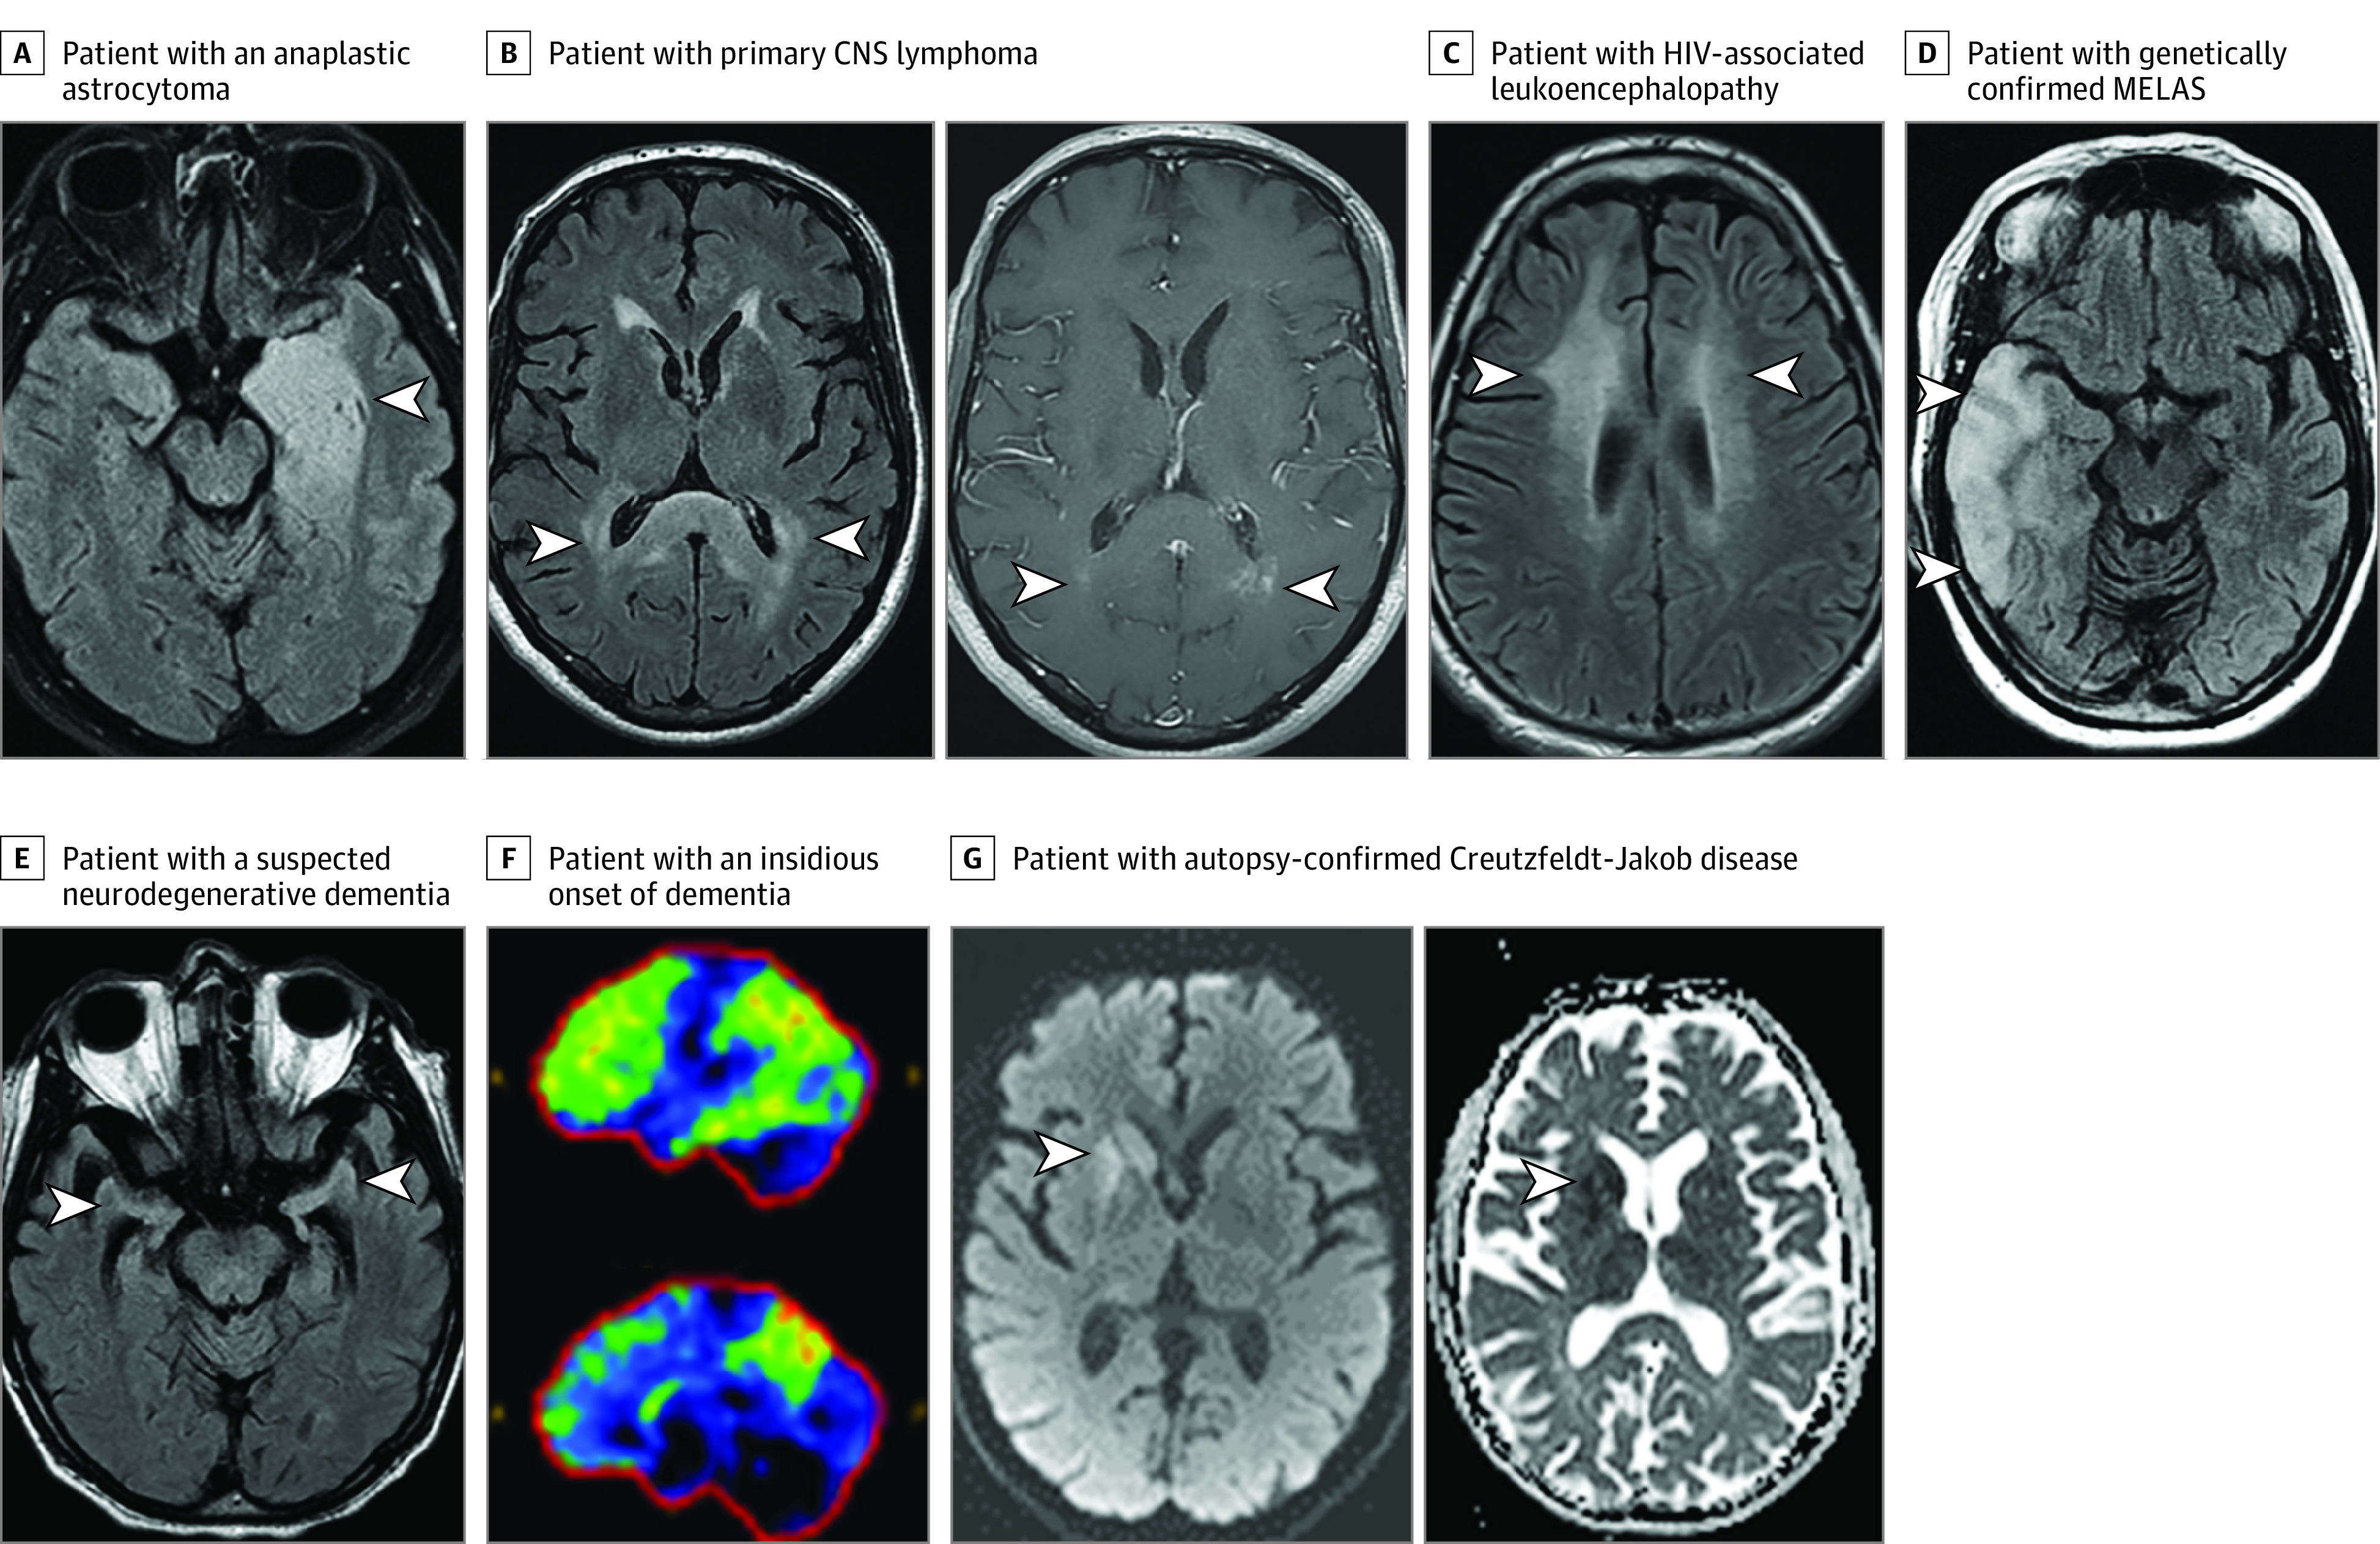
Imaging Examples of Patients Who Were Initially Thought to Have Autoimmune Encephalitis but Later Had an Alternative Diagnosis Made A T2-weighted axial fluid-attenuated inversion recovery (T2-FLAIR) image reveals a left mesial temporal lobe T2-hyperintensity and swelling (A, arrowhead) in a patient with an anaplastic astrocytoma. Note in retrospect the fullness/enlargement of the affected region, possibly suggesting some mass effect. Axial T2-FLAIR image reveals bilateral splenium T2-hyperintensity (B, left panel, arrowheads) with multifocal punctate enhancement (B, right panel, arrowheads) in a patient with primary central nervous system (CNS) lymphoma. An axial T2-FLAIR image reveals bilateral confluent T2-hyperintensity in the subcortical white matter (C, arrowheads) in a patient with HIV-associated leukoencephalopathy. Axial T2-FLAIR image reveals right temporal cortical swelling and T2-hyperintensity (D, arrowheads) in a patient with genetically confirmed mitochondrial encephalomyopathy, lactic acidosis, and stroke-like episodes (MELAS). An axial T2-FLAIR image shows disproportionate bilateral hippocampal atrophy (E, arrowheads) in a patient with a suspected neurodegenerative dementia with features potentially consistent with mixed Alzheimer disease and dementia with Lewy bodies. ^18^F-Fluorodeoxyglucose positron emission tomography reveals reduced uptake of glucose (normal, dark blue/black; mildly reduced, green; moderately reduced, yellow; severely reduced, red) in the frontotemporoparietal region, precuneus and posterior cingulate (F) most suspicious for underlying Alzheimer disease in a patient with an insidious onset of dementia and elevated cerebrospinal fluid phospho-Tau and low cerebrospinal fluid amyloid-β 42 also suggestive of this diagnosis. Axial diffusion weighted hyperintensity (G, left panel) and apparent diffusion coefficient hypointensity (G, right panel) consistent with restricted diffusion in the right caudate and putamen in a patient in whom autopsy later confirmed Creutzfeldt-Jakob disease.
